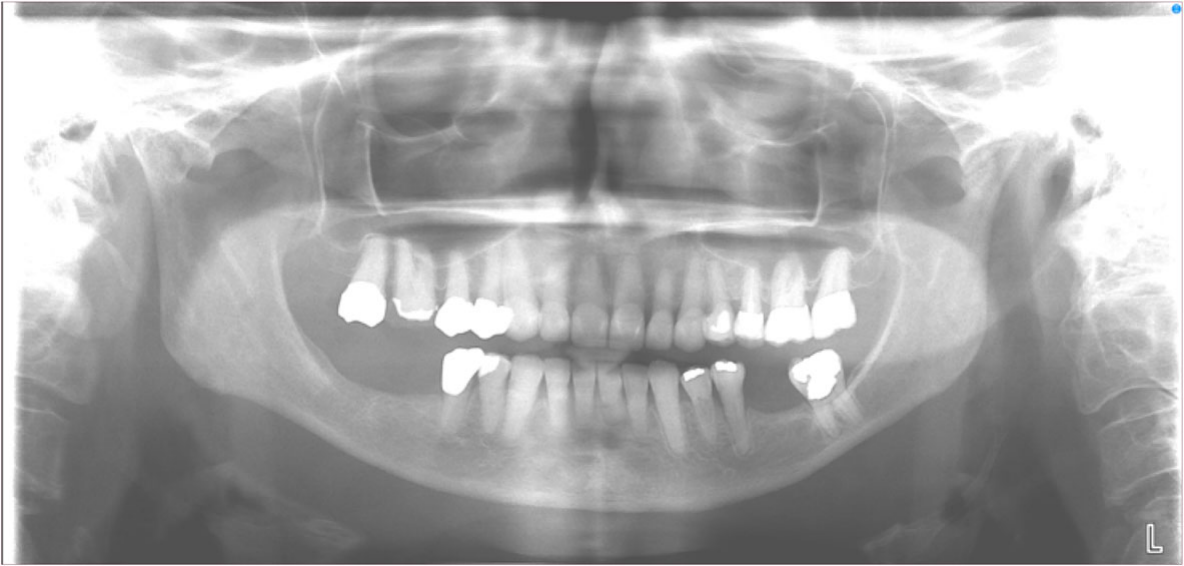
Panoramic radiograph taken 15 months prior to initial CBCT study. The brightness has been increased to highlight soft tissue anatomy. The lesion is not immediately apparent on the radiograph
